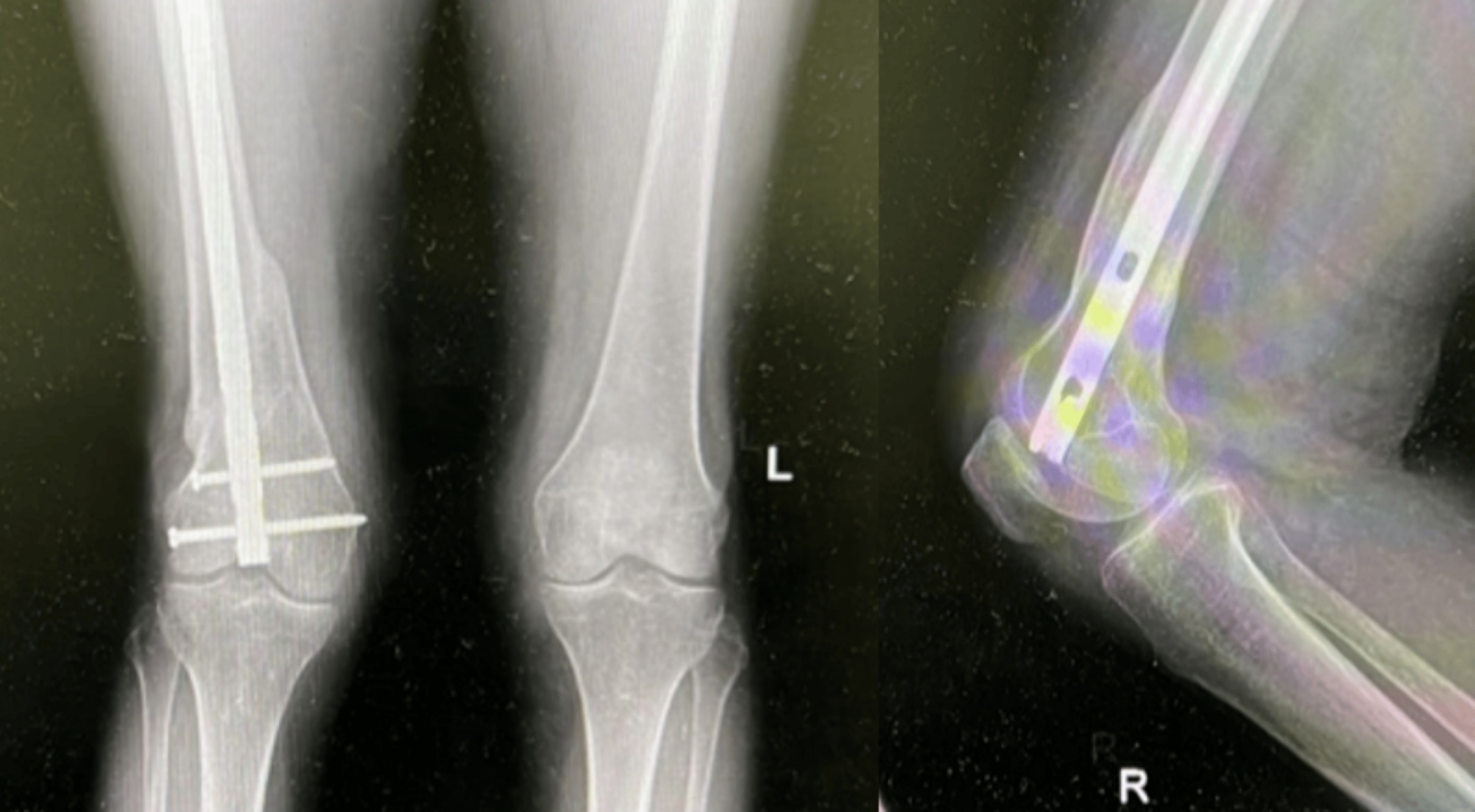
Case 6 X-ray showing complete union of the DFF at eight months DFF: distal femoral fracture
